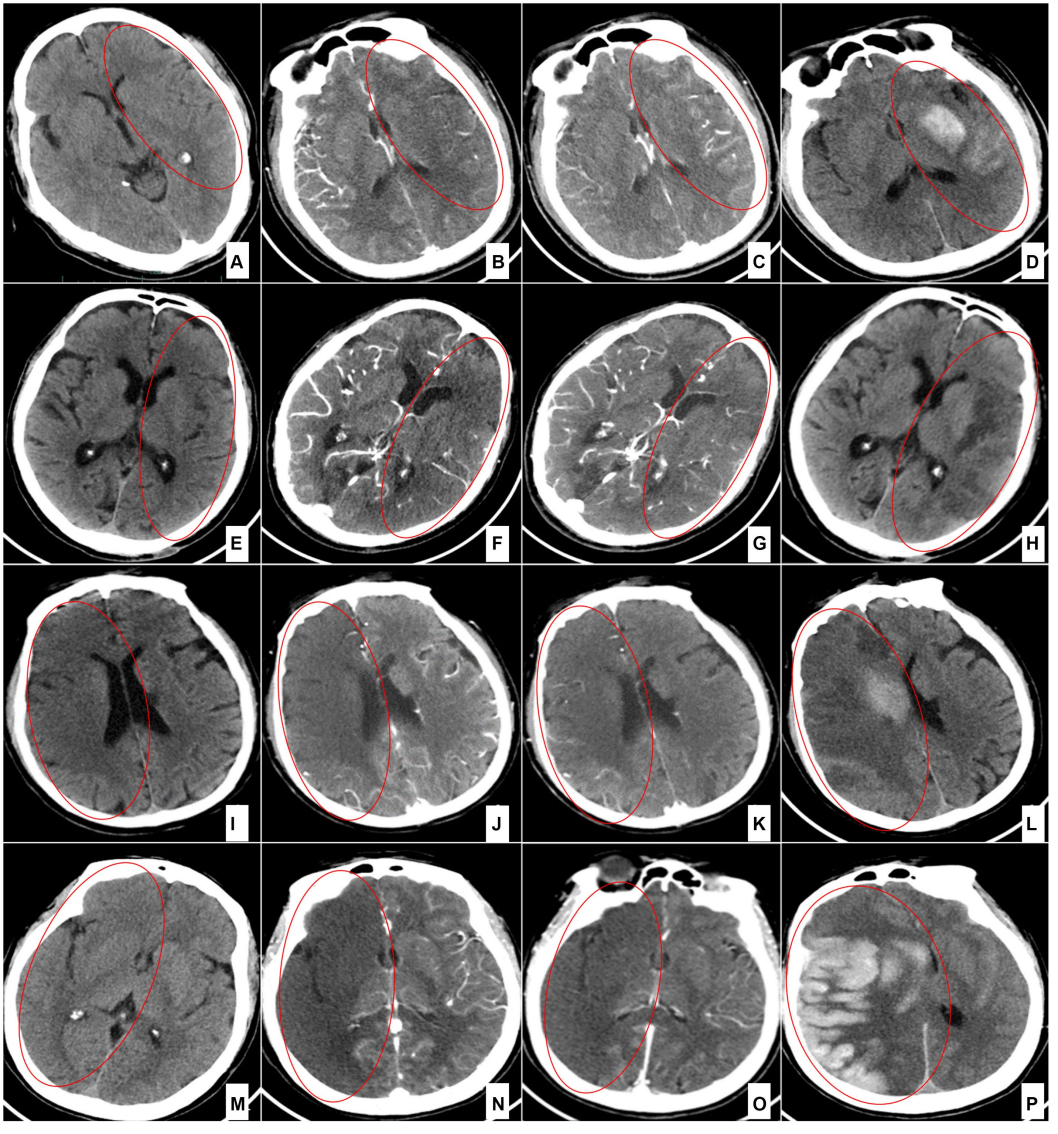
Collateral circulation grading for each category of the 4-point scale. (A–D) Grade 3 (100% filling of the occluded territory). A case of left middle cerebral artery occlusion 4 h after onset: preoperative NCCT (A); arterial phase of the CTA source image (B); delayed phase of the CTA source image (C); and NCCT (D) 24 h after the operation. (E–H) Grade 2 (>50 and < 100% filling of the occluded territory). A case of left middle cerebral artery occlusion 4 h after onset: preoperative NCCT (E); arterial phase of the CTA source image (F); delayed phase of the CTA source image (G); and NCCT (H) 24 h after the operation. (I–L) Grade 1 (>0% and ≤ 50% filling of the occluded territory). A case of terminal occlusion of the right internal carotid artery 2 h after onset: preoperative NCCT (I); arterial phase of the CTA source image (J); delayed phase of the CTA source image (K); and NCCT (L) 8 h after the operation. (M–P) Grade 0 (0% filling of the occluded territory). A case of right internal carotid artery occlusion 1 h after onset: preoperative NCCT (M); arterial phase of the CTA source image (N); delayed phase of the CTA source image (O); and NCCT (P) 6 h after the operation.
